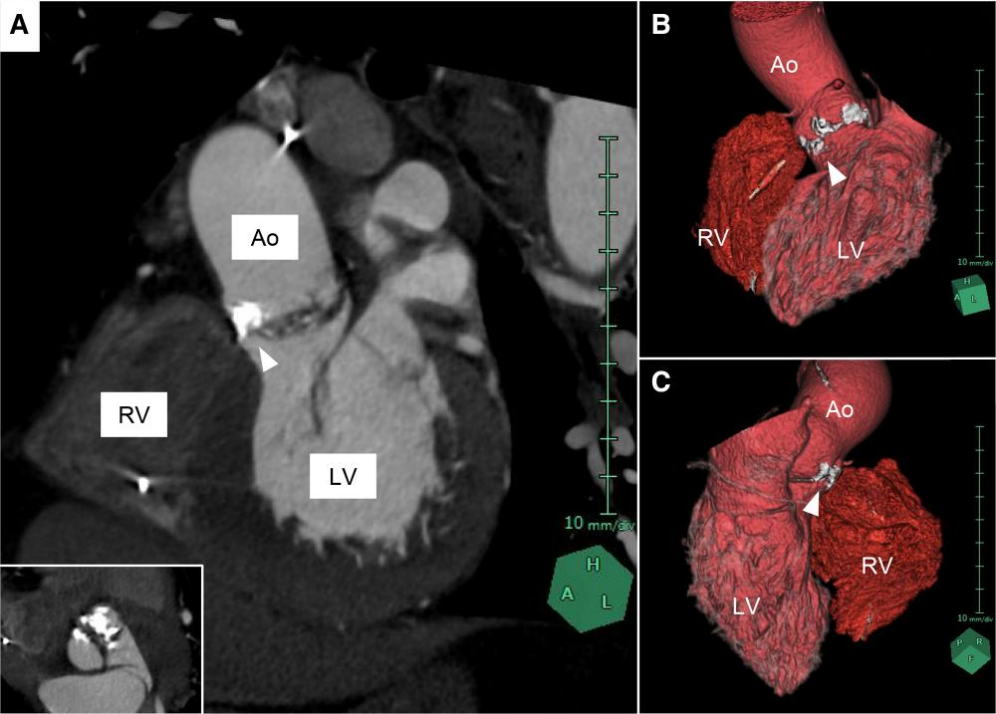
Calcified aortic valve complex on computed tomography. (*A*) Left anterior oblique cross-sectional image reveals heavy aortic valve calcification extending to the interventricular membranous septum. Inset image shows a view parallel to the aortic valve. (*B* and *C*) 3D images reconstructed from computed tomography. White arrowheads indicate calcification attached to aortic valve. Ao, ascending aorta; LV, left ventricle; RV, right ventricle.
